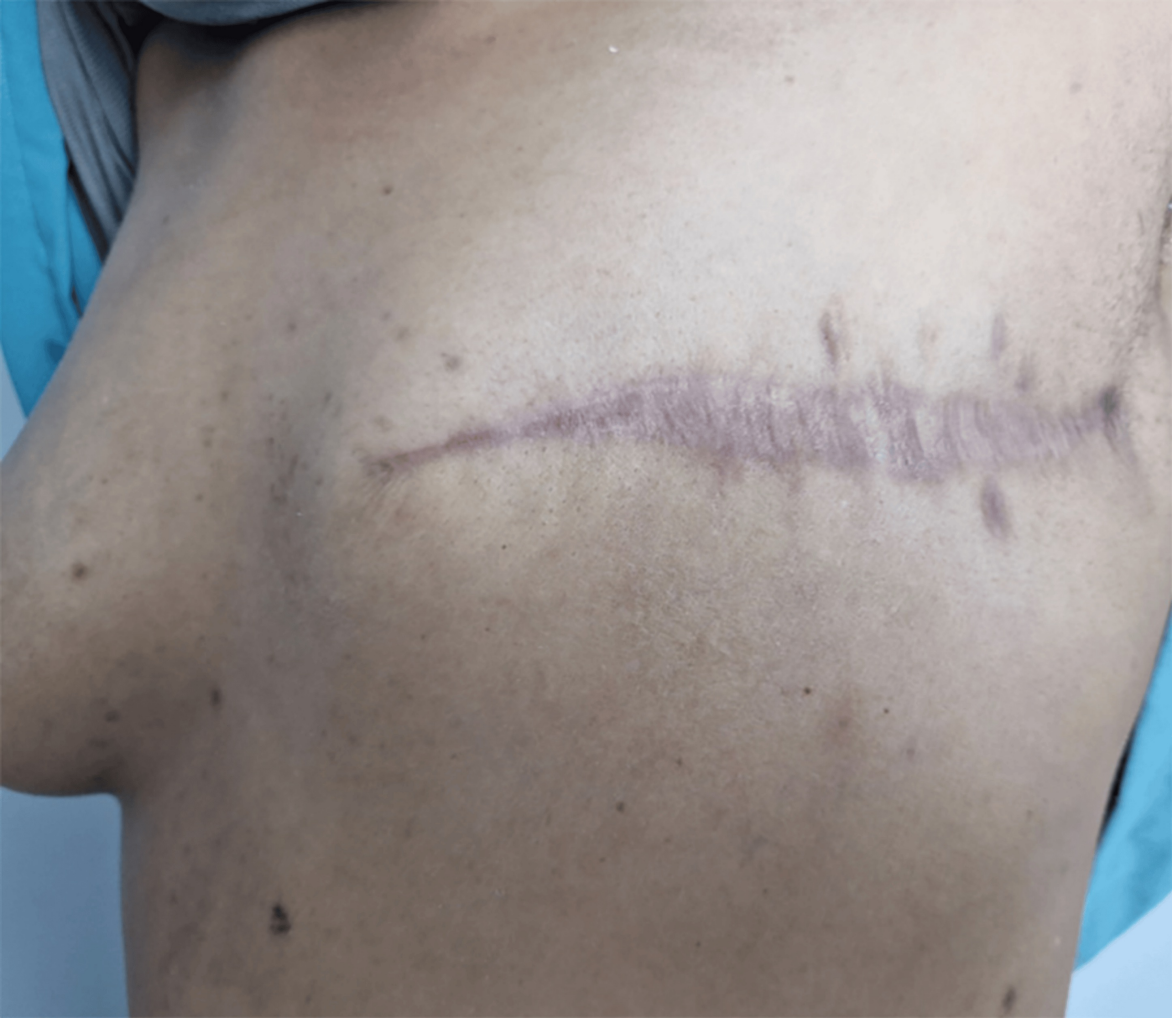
Clinical image after mastectomy (first case). Clean scar with no signs of recurrence on follow-up ultrasound.
